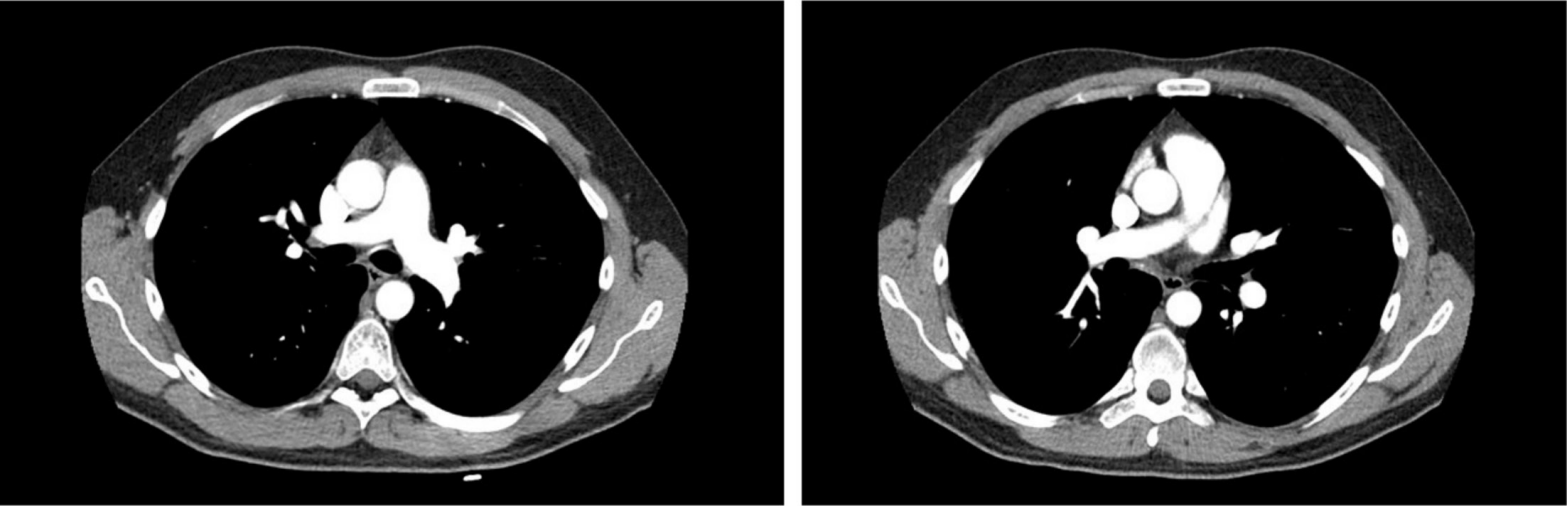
Thoracic CT angiography, 3 months after discharge: Complete resolution of thrombosis and signs of pulmonary infarction.
